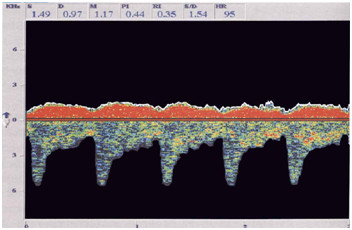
An example of an examination of the arteriovenous cerebral balance with ultrasound dopplerography[30]. If we do not take into account the degree of violation of this balance, treatment of any vascular pathology in the regional reservoir may be not effective.
